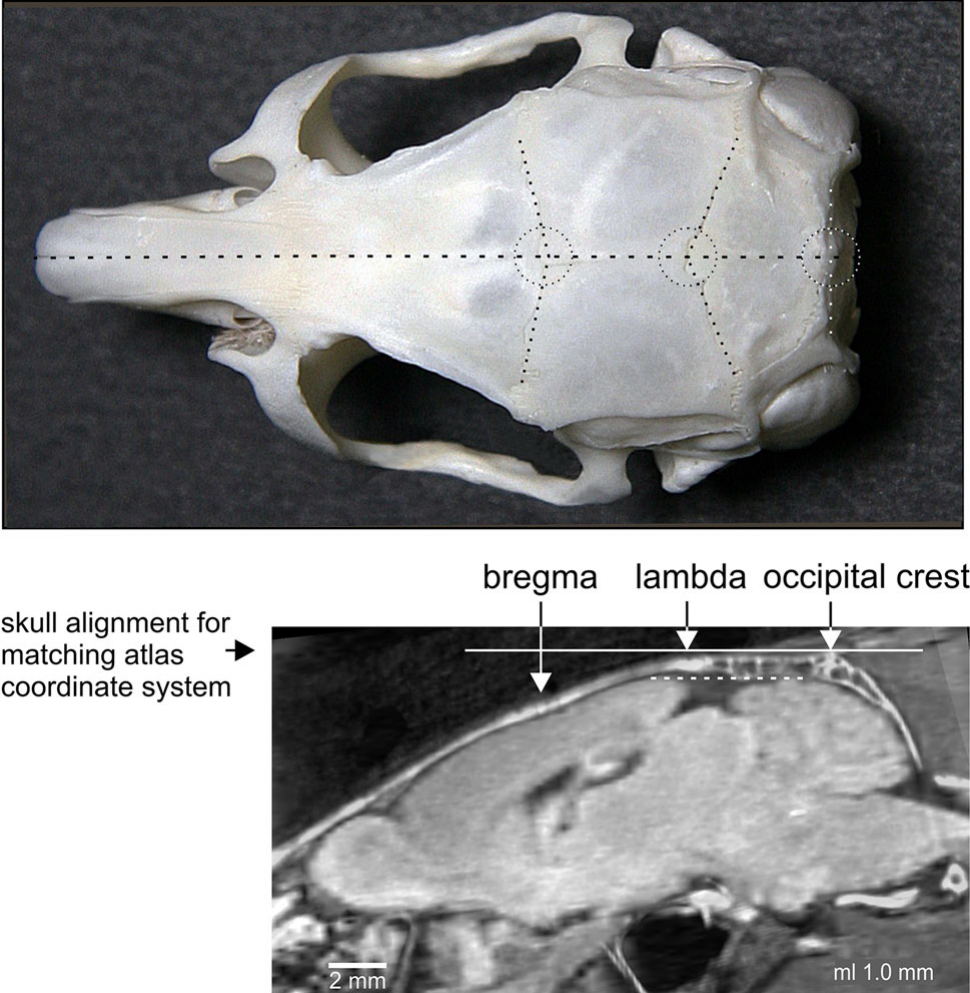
Atlas coordinate system and stereotaxic reference system. *Upper panel* landmarks bregma, lambda and occipital crest (*encircled*) on the skull of a Mongolian gerbil in a *top view*. They are defined by the intersection of lines (*dotted lines*) approximating the course of the bone sutures. *Lower panel* montage of CT skull image and MR brain image at a parasagittal distance of 1 mm in standard orientation of the atlas. The *solid line* corresponds to the horizontal plane through lambda and occipital crest, which is parallel to the plane through the highest points of cerebrum and cerebellum (*dotted line*)
